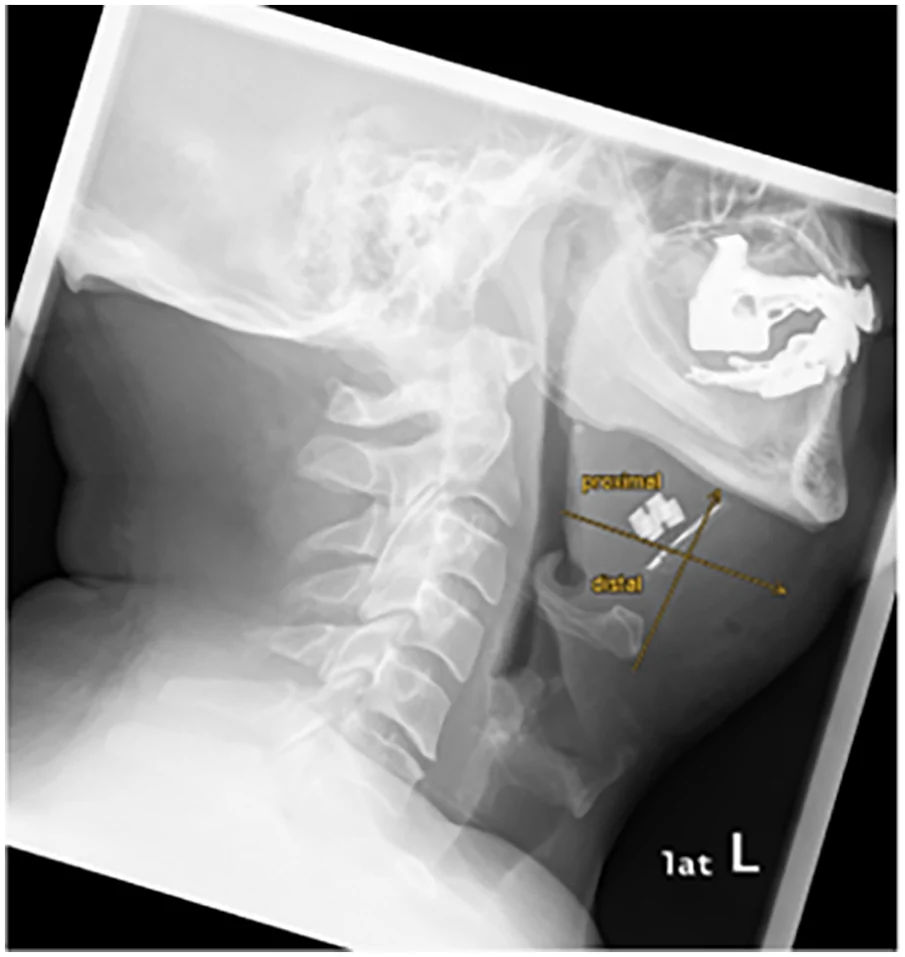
x-ray (lateral). Position of paddle electronics in relation to the mandible.
